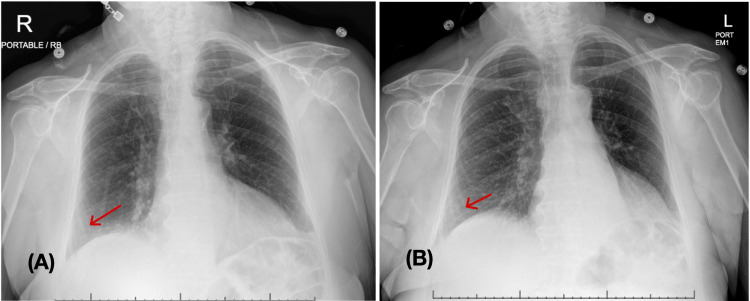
Chest x-ray anterior and posterior (AP) view (A) Left: Prior admission 10 days ago - subtle opacity at the right lung base; (B) Right: latest admission - right basilar opacities have slightly improved, no other significant interval change
